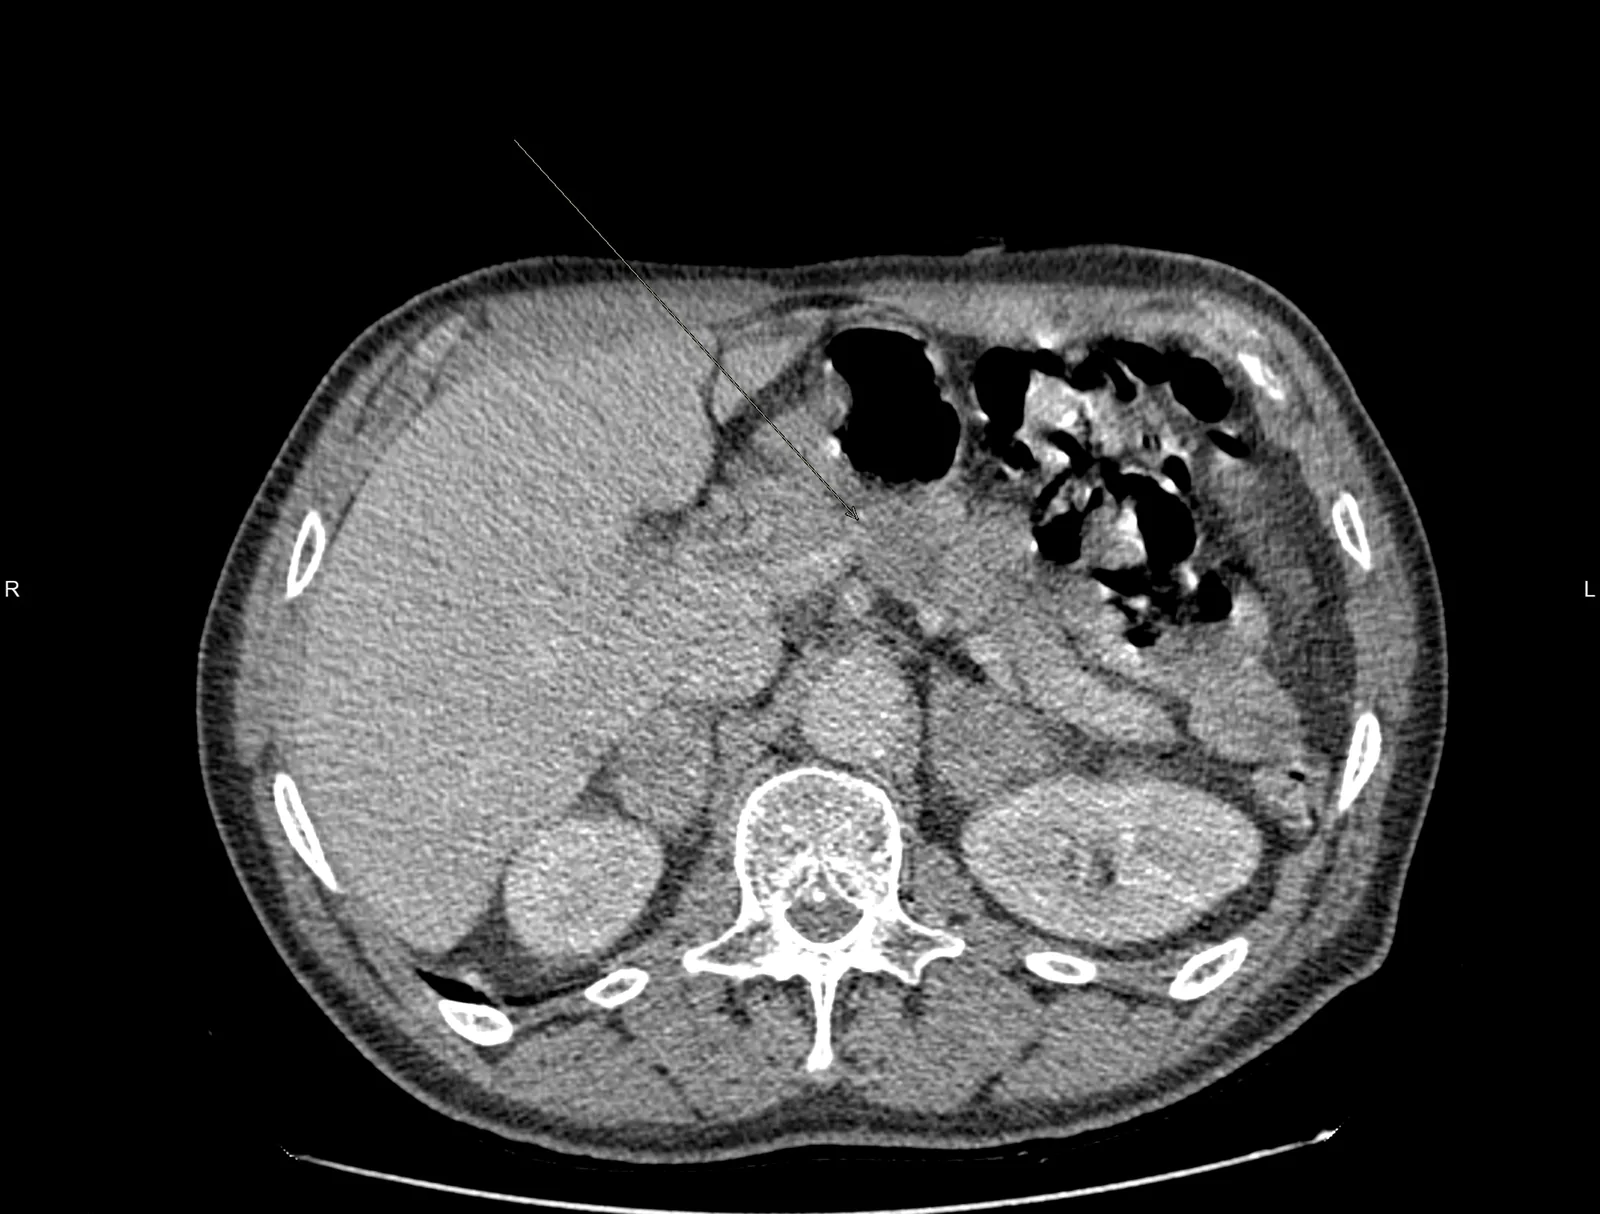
Abdominal CT demonstrating an ill-defined pancreatic body lesion Axial CT of the abdomen demonstrates an ill-defined hypodense lesion within the body of the pancreas. The arrow indicates the pancreatic lesion, measuring approximately 1.0 × 2.0 cm. Image interpretation is limited by motion artifact during image acquisition CT: computed tomography
